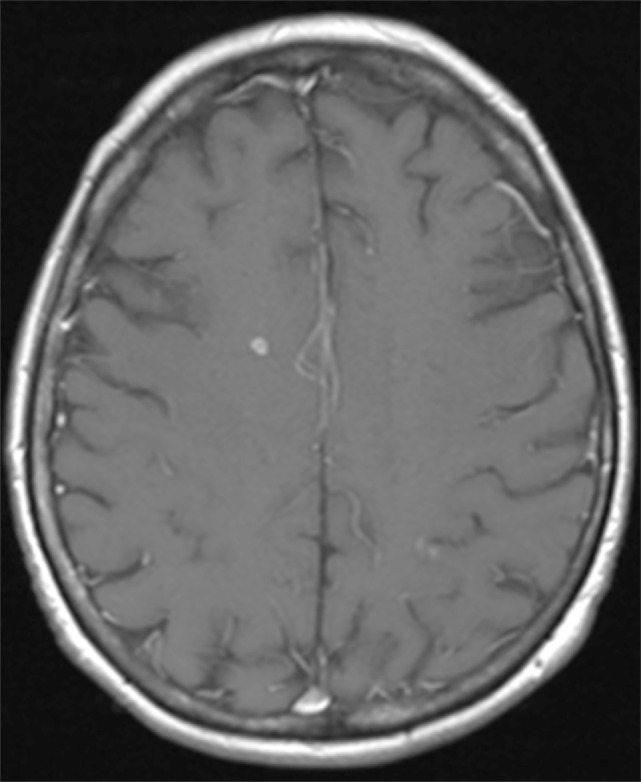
Brain magnestic resonance imaging in March 2014. T1-weighted image after gadolinium of the brain shows a small right frontal enhancing cerebral lesion.
